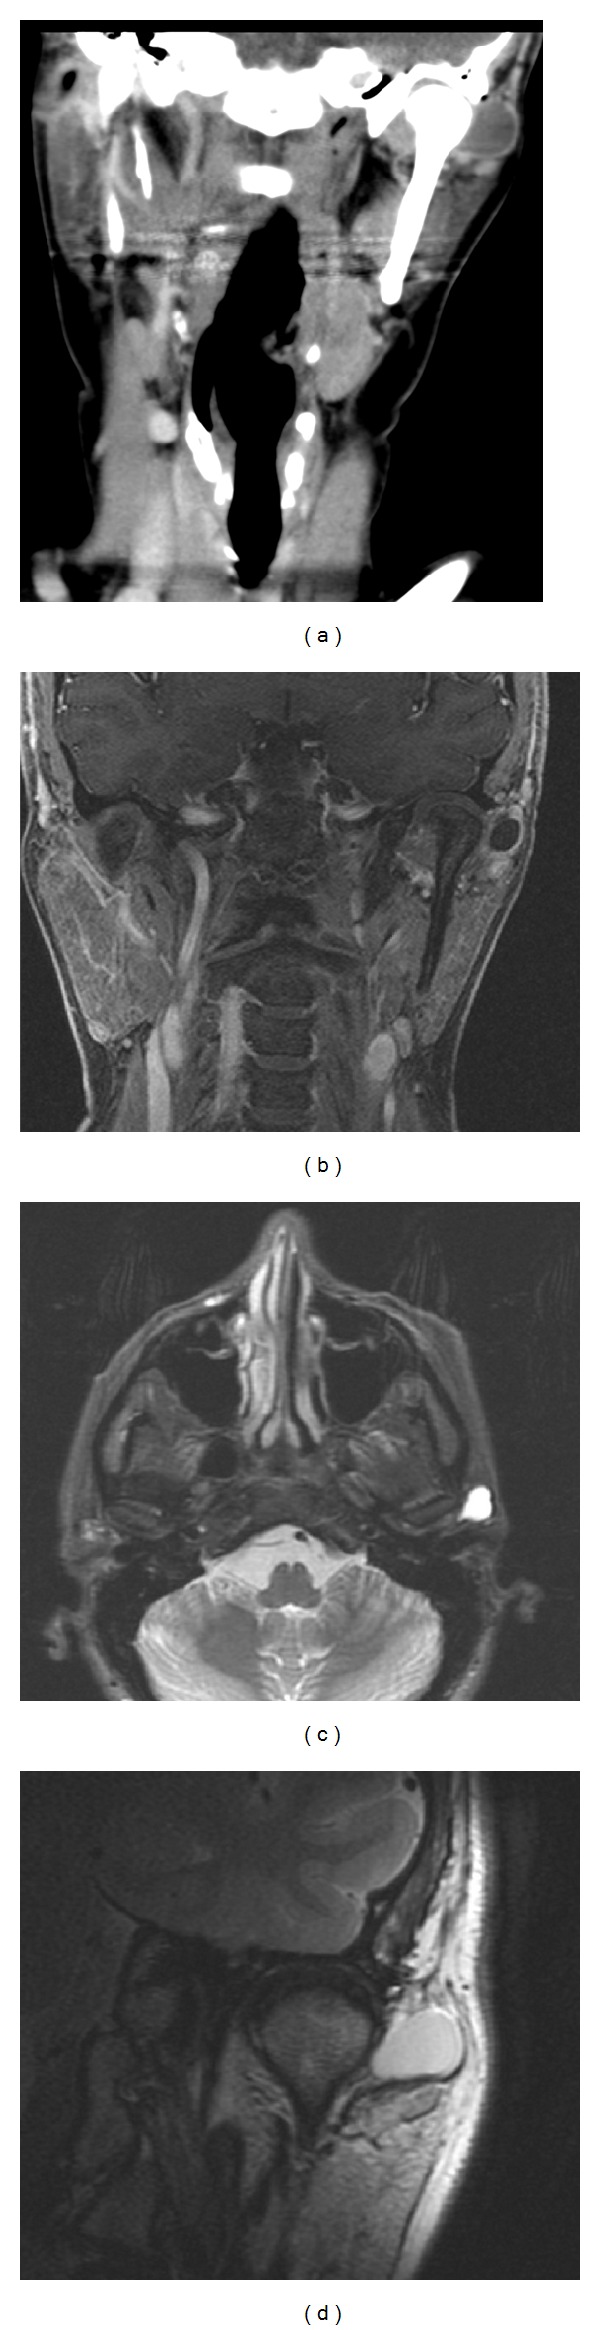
Imaging findings based on the coronal reconstruction of CT (a) and MRI sections (coronal with Gd-DTPA (b), axial STIR (c), and coronal FSE-T2 (d)) showing the features of a cystic lesion adjacent to the left TMJ.
